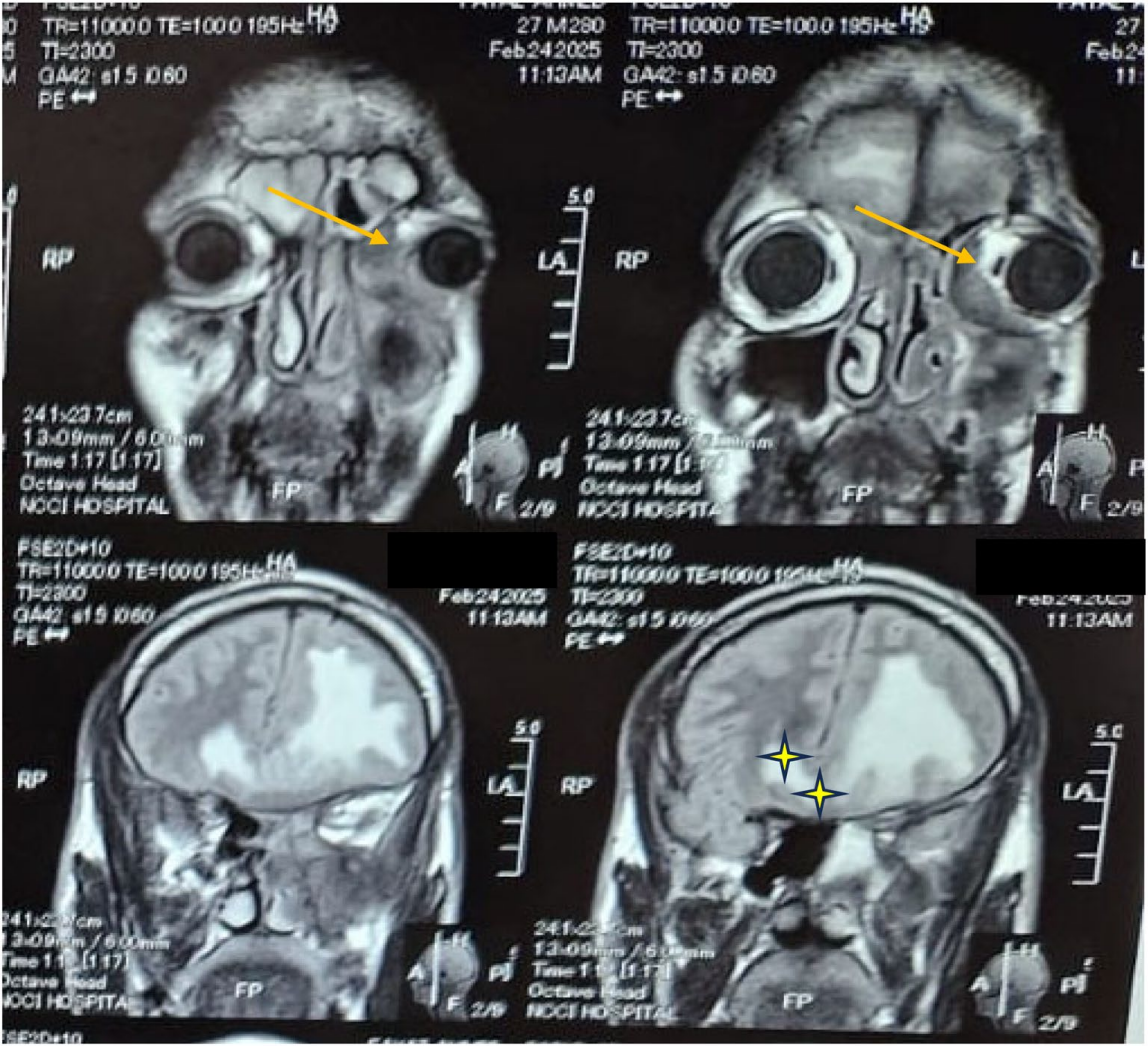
Magnetic resonance imaging (MRI) revealing midline shift to the right (asterisk) and lateral displacement of the left globe.
